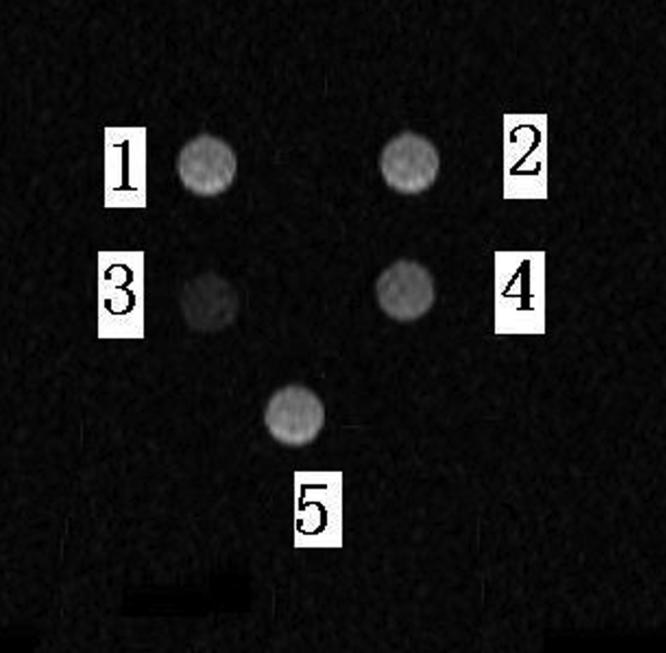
Magnetic resonance image of c-erbB2 antisense probe transfected SK-Br-3 cells. Transfected and untransfected cells, a probe culture medium, a no-probe culture medium, and distilled water were placed into Ependorf tubes and scanned using MR transactions. 1: untransfected cells, 2: no-probe culture medium, 3: transfected cells, 4: probe culture medium, 5: distilled water. From the figure, we can observe that c-erbB2 antisence probe can improve the magnetic properties of SK-Br-3 cells and decrease the signal intensity in MR scanning obviously.
